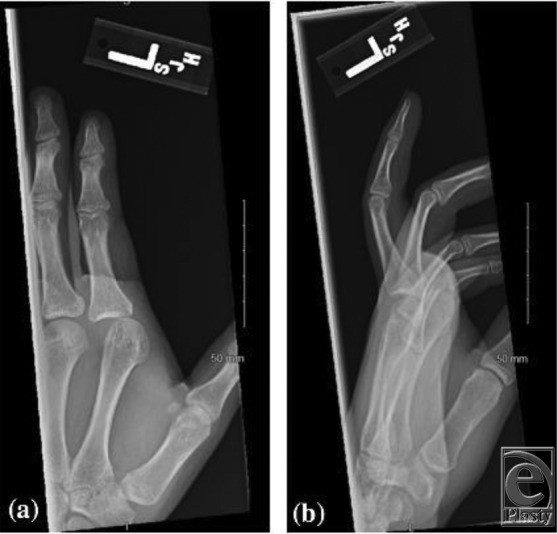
Posteroanterior (*a*) and lateral (*b*) radiographic images showing dorsal dislocation of the left index metacarpophalangeal joint and ulnar shift of the proximal phalanx.
